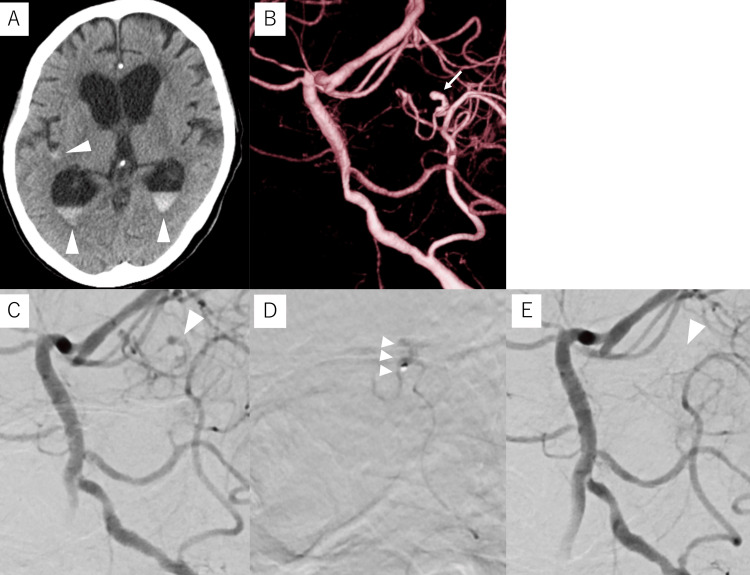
Preoperative and intraoperative imaging of Case 5 Case 5: A 78-year-old female with intraventricular hemorrhage and subarachnoid hemorrhage in the Sylvian fissure. (A) Computed tomography (CT) upon admission shows an intraventricular hemorrhage and subarachnoid hemorrhage in the Sylvian fissure (arrowheads). (B) Digital subtraction angiography (DSA) of the left vertebral artery shows a 1.6-mm fusiform aneurysm (arrow) on an inferior vermian branch of the posterior inferior cerebellar artery. (C) Selective angiography of the parent artery (working-angle view) shows a fusiform aneurysm (arrowhead). (D) The aneurysm and parent artery are embolized with 20% N-butyl cyanoacrylate glue (arrowheads). (E) Post-embolization DSA of the left vertebral artery shows the disappearance of the aneurysm (arrowhead).
